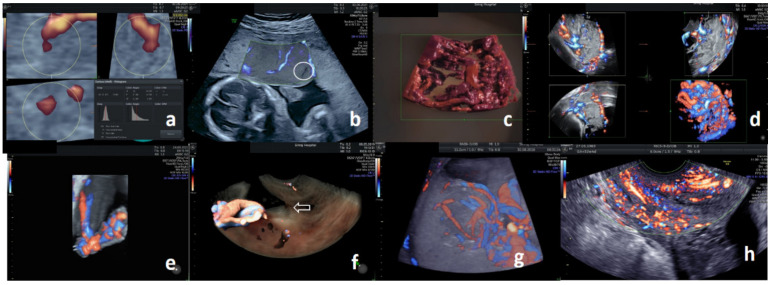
Extra-fetal assessment with three-dimensional sonoangiography. (a) Histogram flow-volume indices of the placenta at 26 weeks of gestation; three-dimensional (3D) power Doppler (PD) with a spherical representation of the entire placental vascular tree. (b) Normal parenchymal vasculatures of the placenta at 24 weeks of gestation; SlowflowHD (GE GmbH, Vienna, Austria). Note the virtual absence of flow in the lake (circle). (c) Confluent parenchymal vasculatures of placenta percreta at 29 weeks of gestation; HDLive Silhouette (GE, Milwaukee, WI, USA), thick-slice, monochrome 3D high-definition flow (HDF). (d) Complicated vasculatures involving the entire thickness of the placenta, with extension to the myometrial–bladder interface of placenta percreta, at 29 weeks of gestation; orthogonal multiplanar 3D HDF. (e) Velamentous umbilical cord insertion at 25 weeks of gestation; thick-slice 3D HDF. Note the transition from coiled umbilical vessels to chorionic vessels with the artery (blue) crossing over the vein (red). (f) Marginal placenta previa at 28 weeks of gestation; HDLive Silhouette (GE, Milwaukee, WI, USA), thick-slice 3D HDF. Note the proximity of the velamentous umbilical cord insertion to the internal cervical os (arrow). (g) Feeding vessels of chorioangioma at 29 weeks of gestation; thick-slice 3D HDF. (h) Parenchymal vasculatures of a normal cervix at 32 weeks of gestation; two-dimensional HDF.
